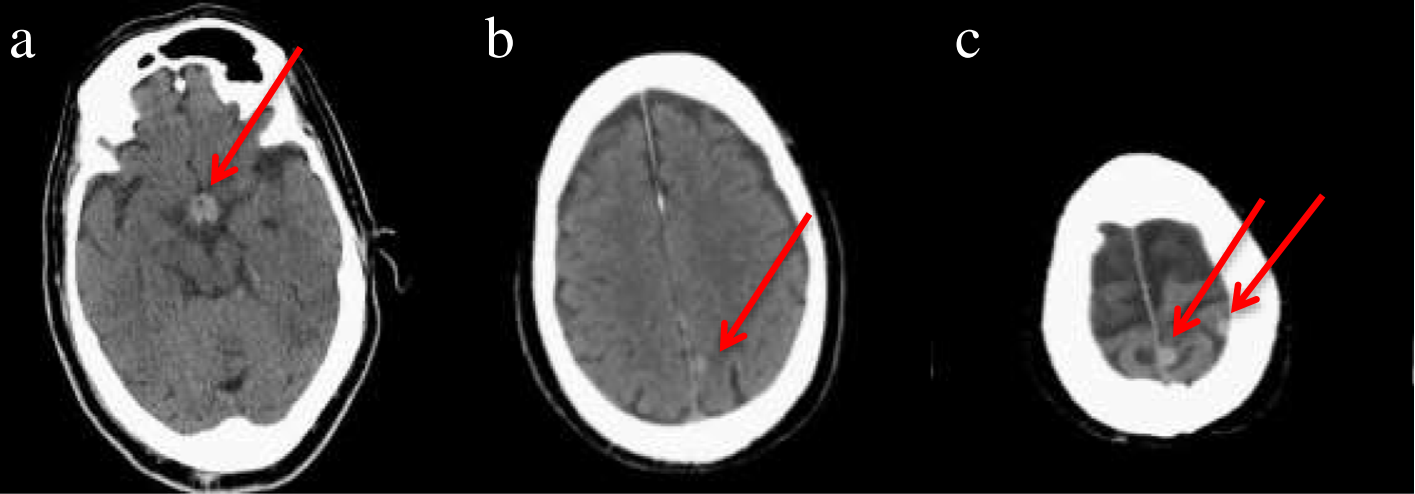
Head computed tomographic scan demonstrating a high-density area on the pituitary (a) and multiple tumor-like lesions pointed to by the arrows (a–c)
